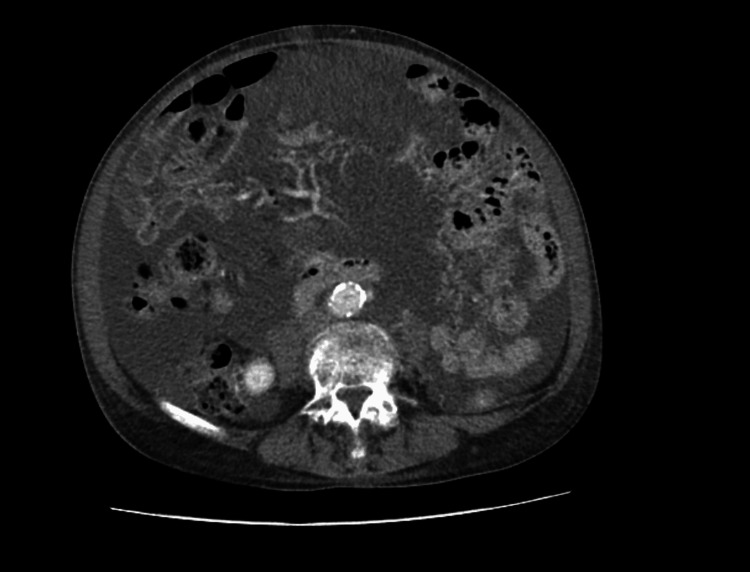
CT abdomen and pelvis with IV contrast showing omental nodularity, cardiophrenic lymphadenopathy, and diffuse intra-abdominal free fluid, consistent with peritoneal disease progression.
